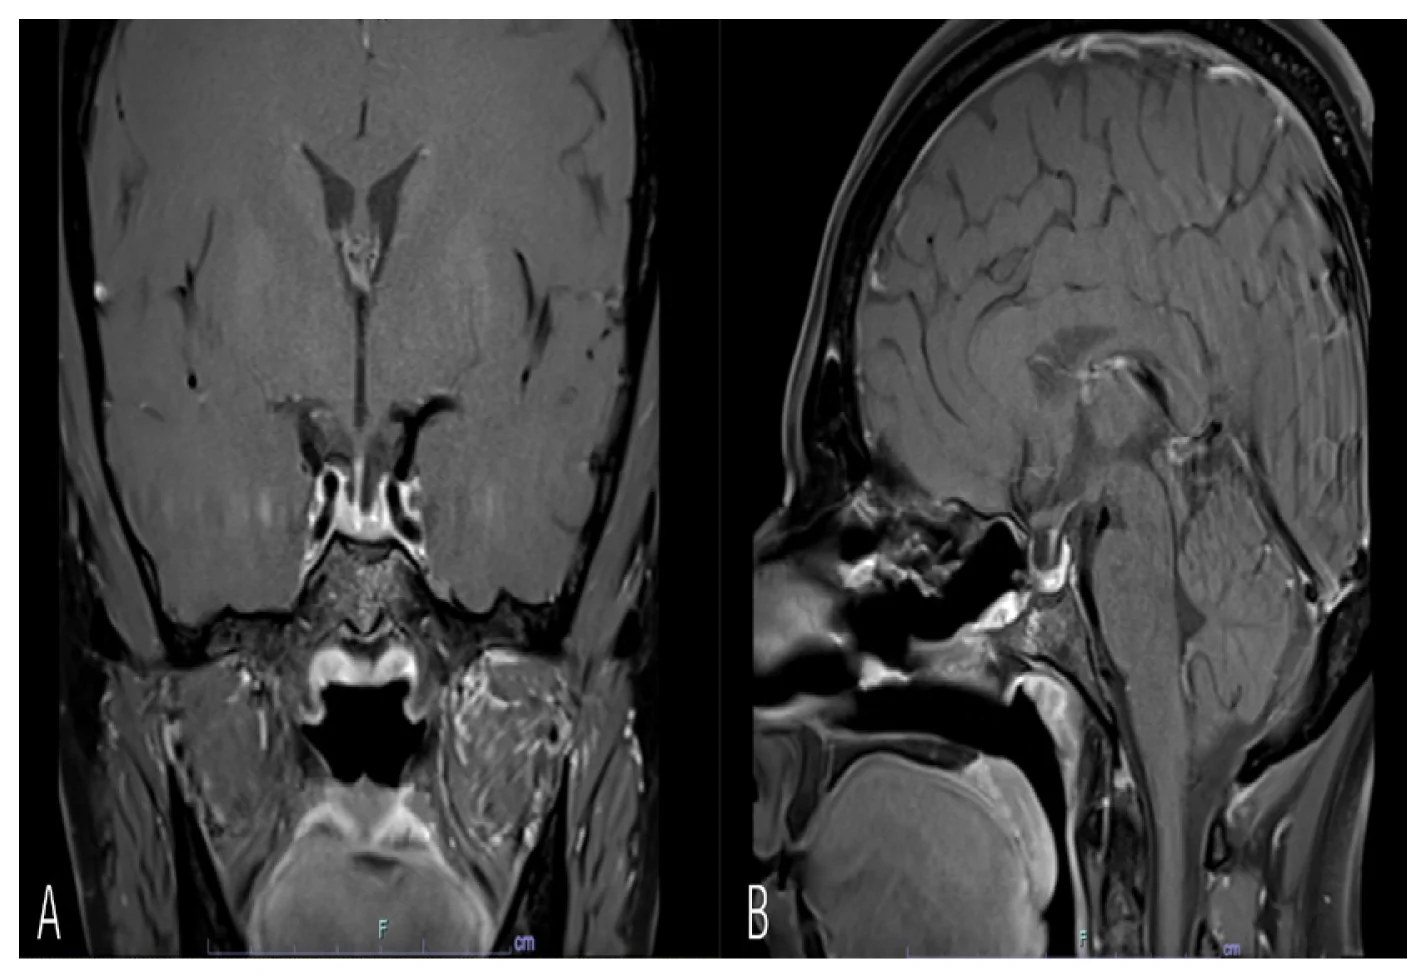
Post-operative T1W magnetic resonance imaging scan with contrast in the (A) coronal and (B) sagittal view showing no obvious residual.
